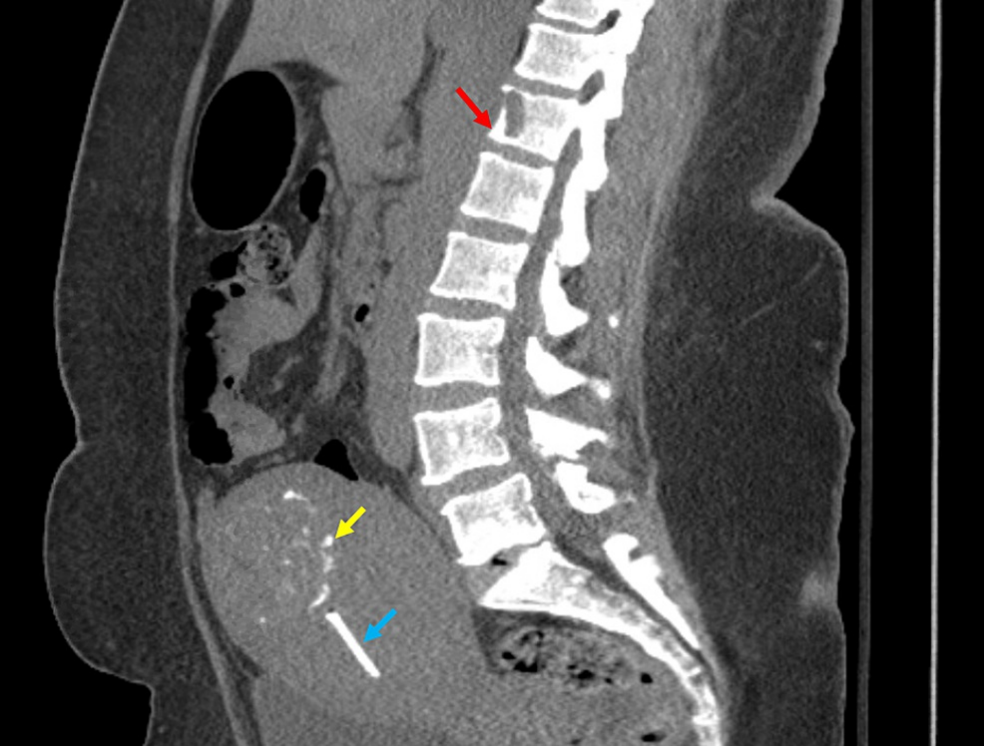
CT scan showing T6 osteolytic lesion (red arrow), calcified fibroid on the uterus (yellow arrow), and intrauterine device in place (blue arrow).
